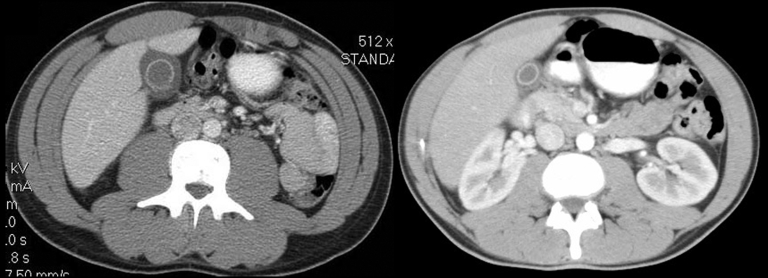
Computed tomographic scans of abdomens of 2 patients with inflammation of the gallbladder.
